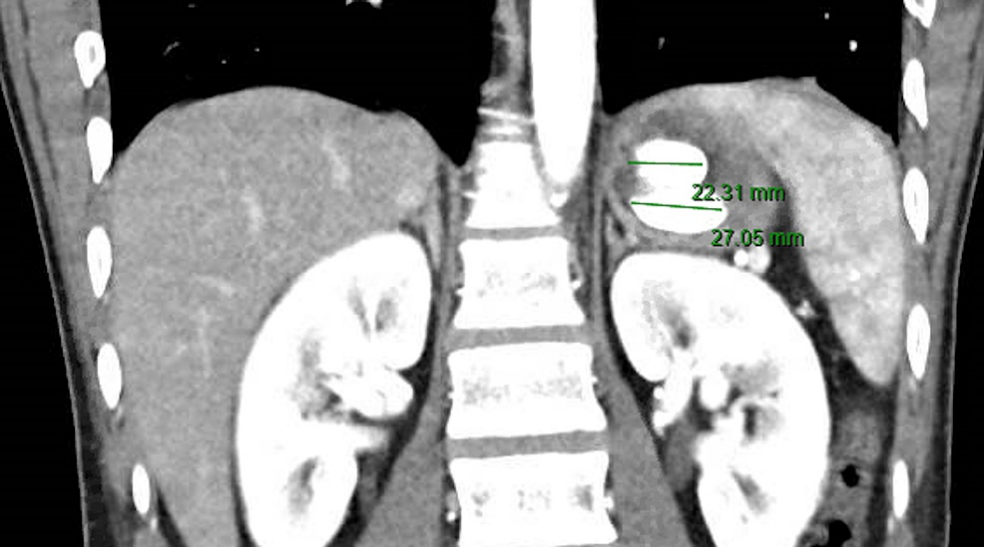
Two foreign bodies seen in the stomach on CT, measured via radiology software CT, computed tomography
